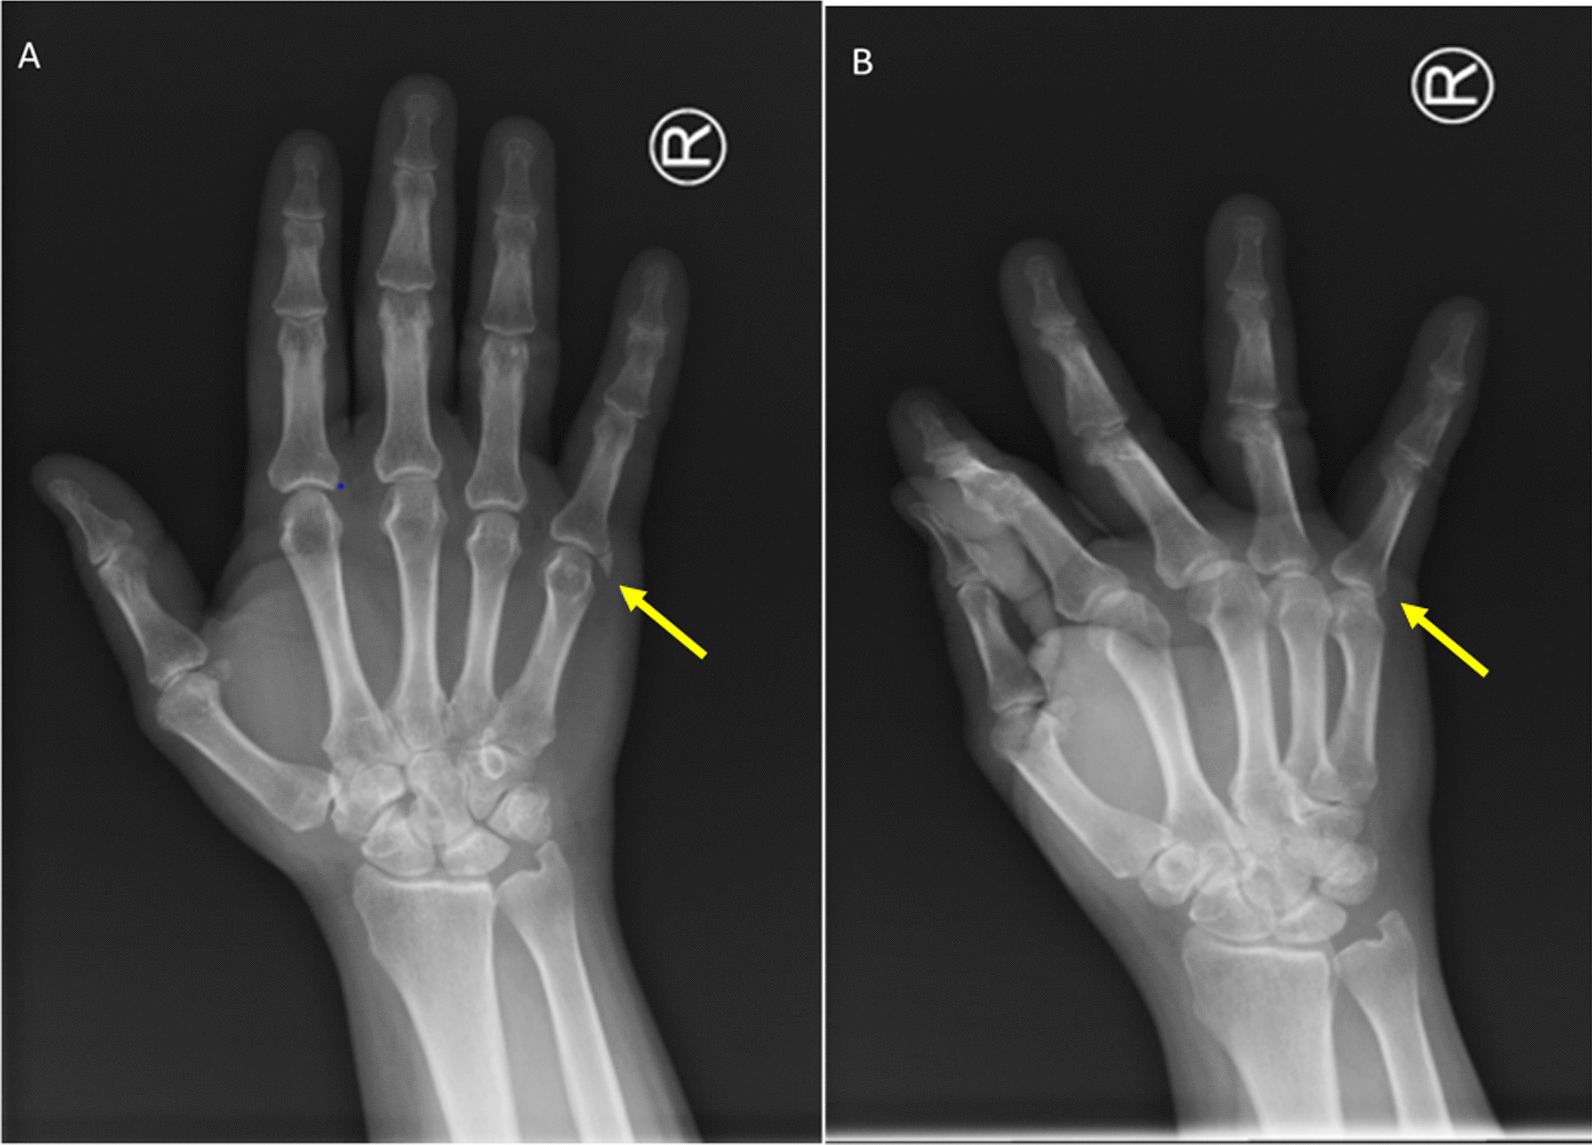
Radiographic imaging showing a calcified lesion concerning for inflammation. A Posterior-anterior view of right hand demonstrating calcification (arrow) adjoining ulnar collateral ligament of fifth metacarpophalangeal joint. B Oblique view providing additional visualization of periarticular calcification (arrow)
